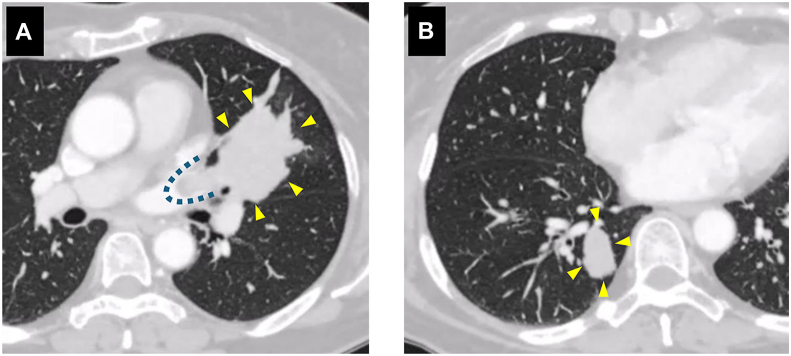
Computed tomography revealed (A) a 5-cm mass in the left upper lobe (yellow arrowheads), with suspected polypoid extension into the left atrium through the left superior pulmonary vein (blue dots), and (B) a 2.5-cm nodule in the right lower lobe (yellow arrowheads).
